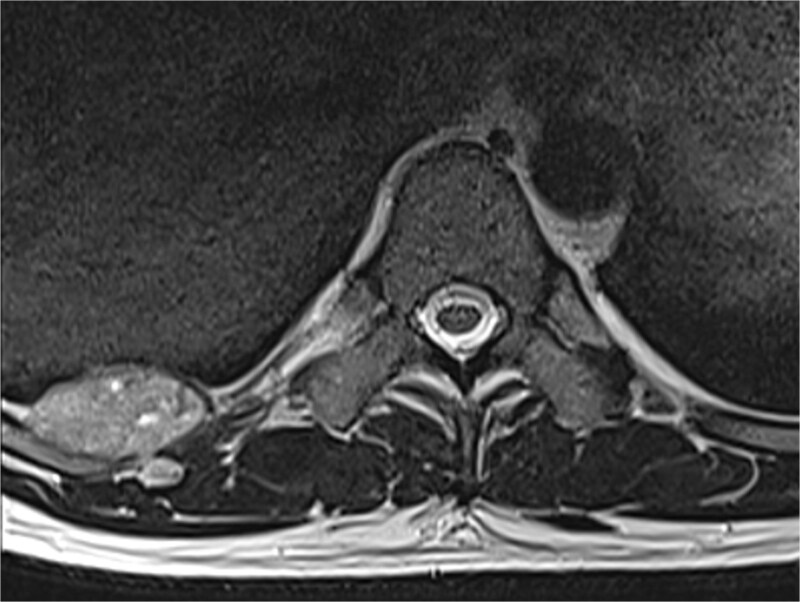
A magnetic resonance imaging scan shows a lesion on the right posterior side of the chest wall.
